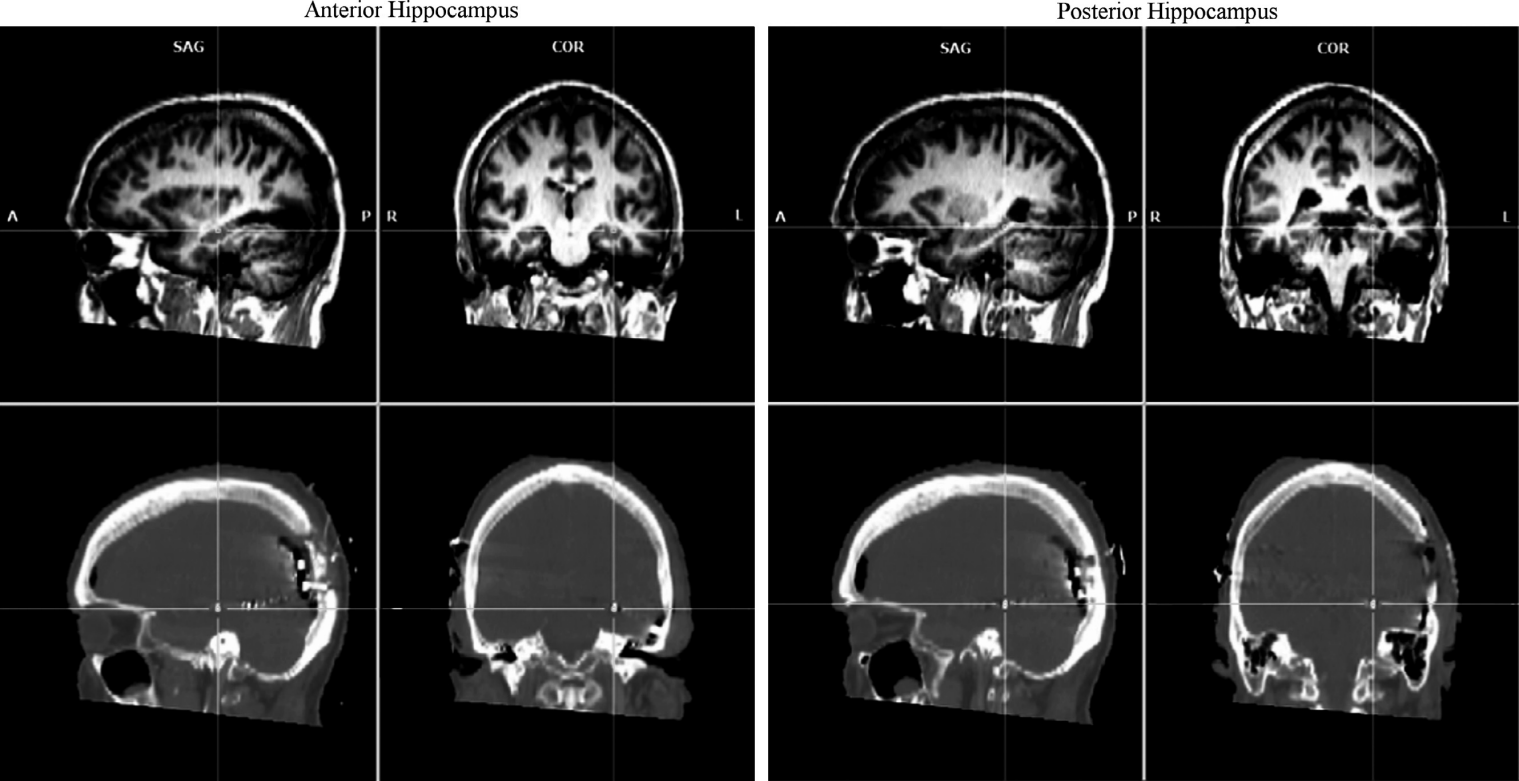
Sagittal (left) and coronal (right) views of MRI (top) and CT (bottom) images of the electrodes position in the anterior and posterior hippocampus.
